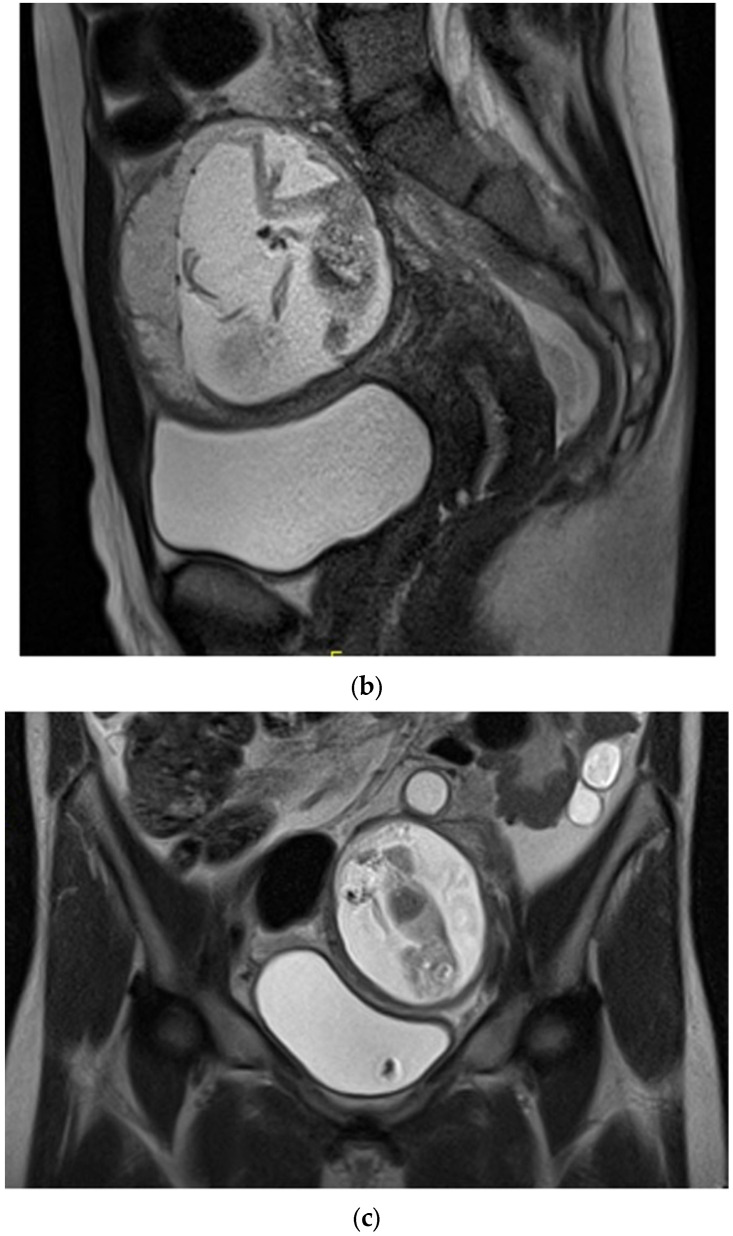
MRI without contrast showed that the ectopic gestational sac exerted mass effect upon the uterus and bladder, and was associated with a moderate amount of pelvic fluid. (a) Axial T2 HASTE demonstrating ectopic pregnancy in the left adnexa with associated mass effect on the bladder and uterus which deviate to the right. The placenta is located anteriorly within the gestational sac. (b) Sagittal T2 HASTE demonstrating ectopic pregnancy located anterior to uterine fundus and superior to bladder. The anterior wall of the gestational sac is located approximately 1.3 cm deep to the overlying skin surface. There is a moderate amount of complex free pelvic fluid. (c) Coronal T2 HASTE demonstrating intact gestational sac superior to the bladder in the left adnexa. The left ovary is partially visualized superior to the gestational sac and contains an incidental 2.0 cm follicle.
